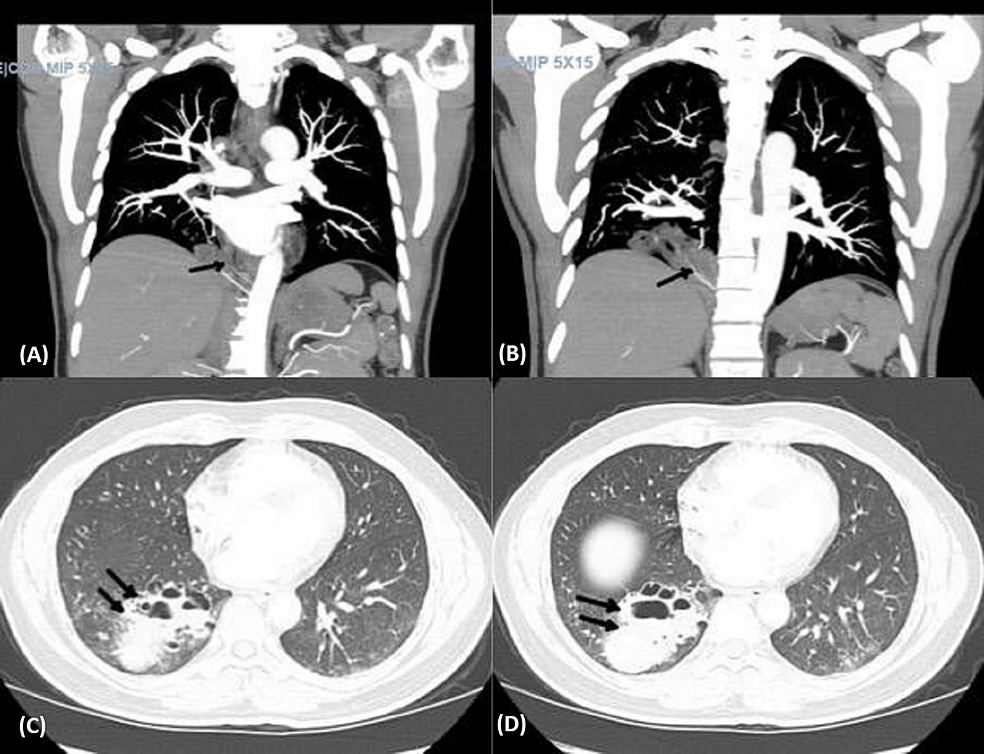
Computed tomography (CT) angiography of the chest showing intralobar pulmonary sequestration at the right lung base with aberrant feeding arteries arising from the lower thoracic aorta (A,B), which is likely the source of acute blood products within this complex blood-filled cavity (C,D).
